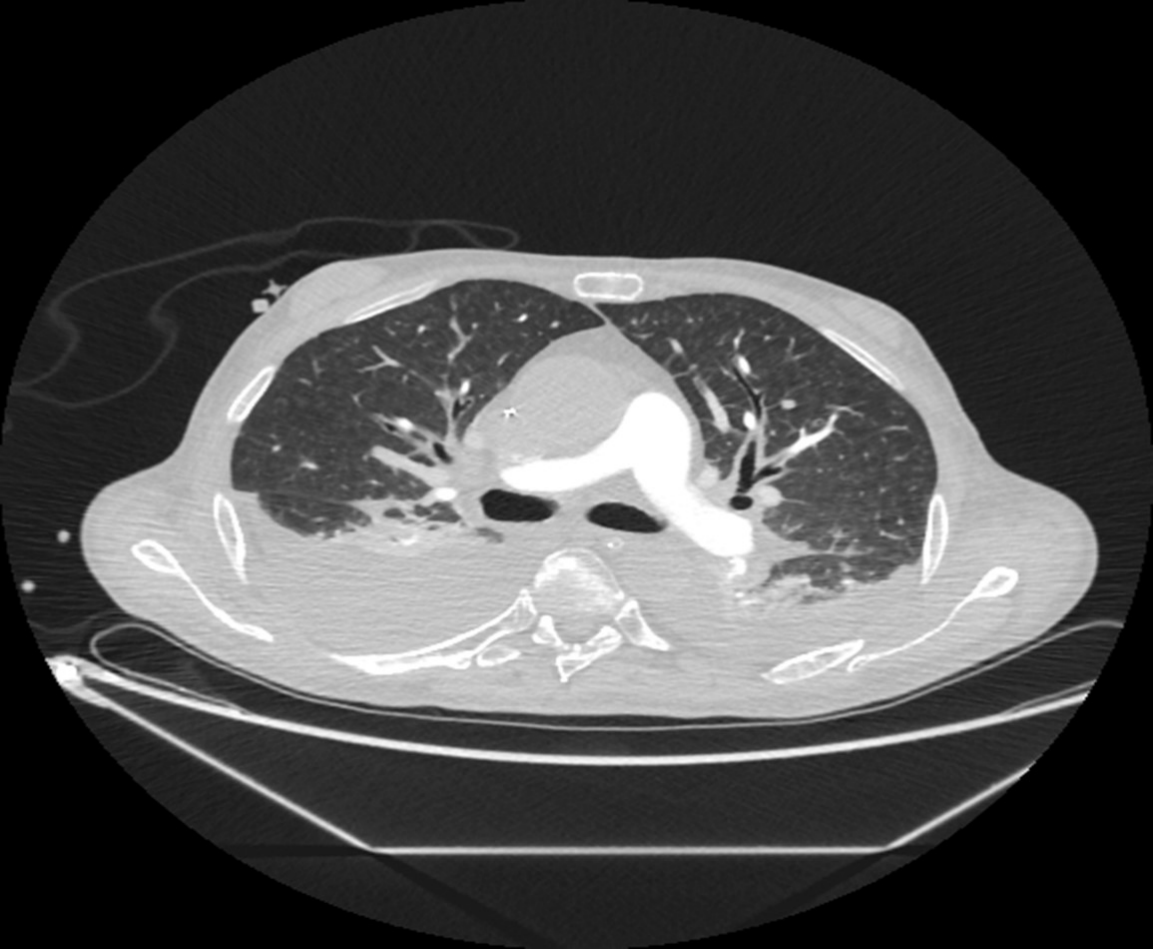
Postcontrast thoracic CT-scan revealing bilateral pleural effusions. No signs for central thromboembolism or pneumothorax were documented.
